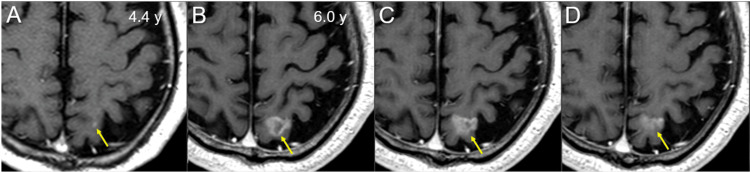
MRI findings at the diagnosis of the brain metastasis The images show CE axial T1-weighted images (WIs); at 4.4 years (y) after the initiation of the first-line chemotherapy; and at 6.0 years (B-D) (A-D) These images are shown at the same magnification and coordinates under co-registration and fusions (A, B: almost the same cross-section; C, D: different cross-sections). (A) A tiny enhancing lesion in the left superior parietal lobule was retrospectively identified. (B-D) A heterogeneously enhancing irregularly-shaped lesion located mainly in the cerebral cortex, with infiltration to the brain surface MRI: magnetic resonance imaging; CE: contrast-enhanced
